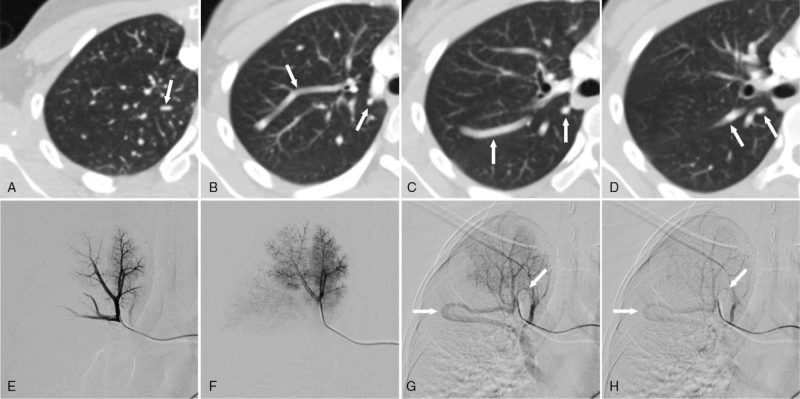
A 23-year-old woman. An abnormal shadow is identified in the right lung. (A)(B)(C)(D) Contrast-enhanced computed tomography shows tortuous vessels that run from the right upper lobe to the lower lobe and are continuous with the right lower pulmonary vein (white arrows). (E)(F)(G)(H) Digital subtraction right pulmonary angiography. (E)(F) Pulmonary arterial phase and lung parenchymal phase. Abnormal vessels are not identified. (G) Early pulmonary venous phase. As normal pulmonary veins are identified, tortuous vessels (white arrows) are also identified. There are two arc-shaped abnormal vessels, which run in and outward from the upper to the middle fields of the right lung. (H) Late pulmonary venous phase. Contrast enhancement in the abnormal vessels (white arrows) is persistent.
